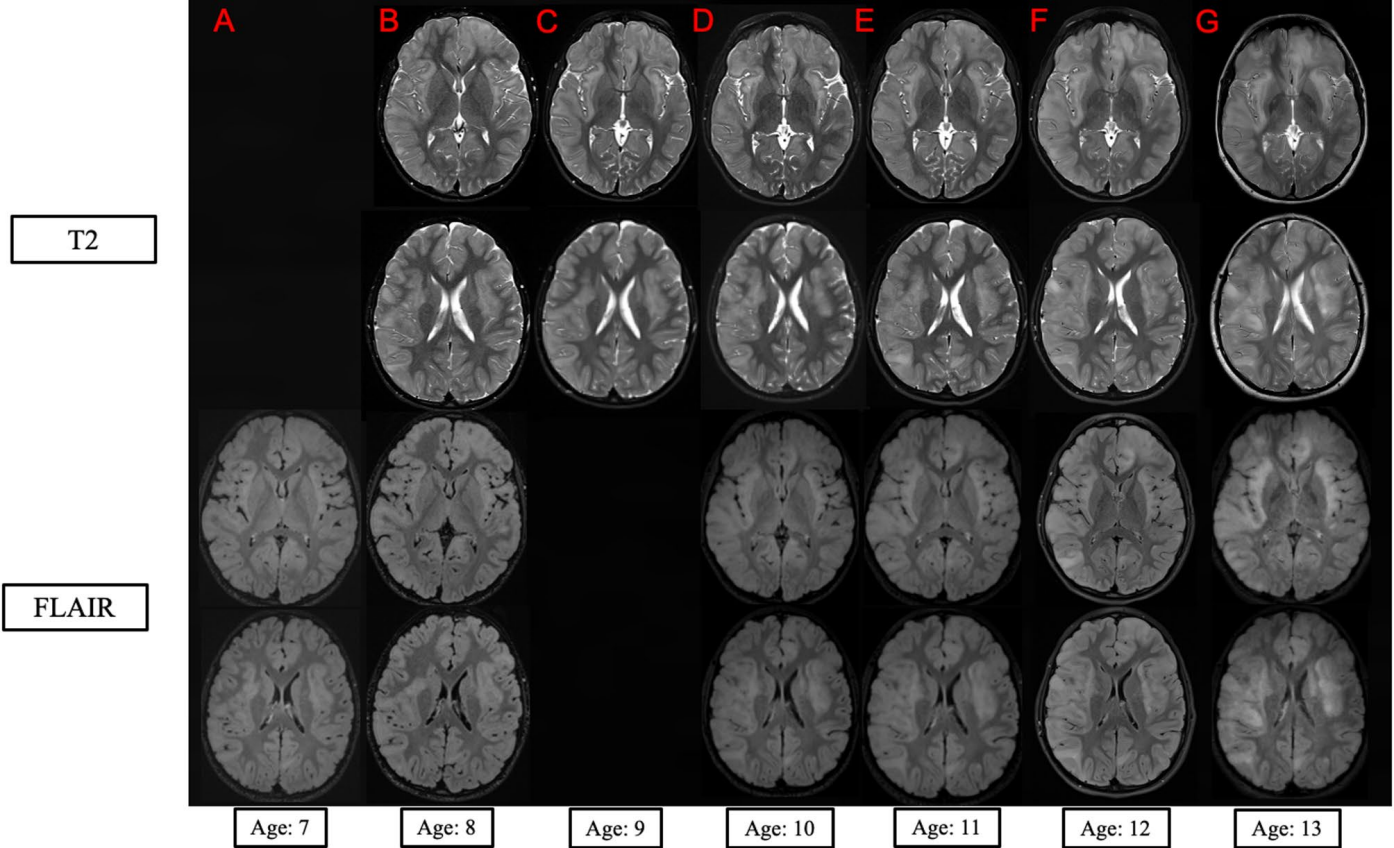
Imaging*. Axial T2 (top two rows) and FLAIR (bottom two rows) MRI sequences at age 7 (A), 8 (B), 9 (C), 10 (D), 11 (E), 12 (F) and age 13 (G) of the patient at the level of insula (top row) and upper lateral ventricles (bottom row), respectively. There is progressive increase in mass effect of the infiltrative tumor throughout the white and gray matter of the insula and both the bilateral frontal and right temporal lobes. There was no Age 7 axial T2 scan, and the Age 9 FLAIR scan was severely motion distorted and not included
